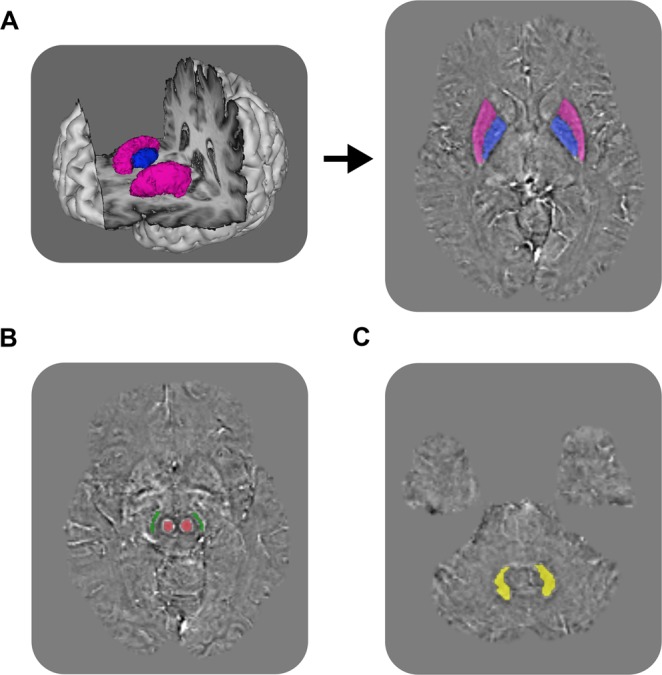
Automated segmentation of globus pallidus (blue) and putamen (magenta) on T1-weighted (A). Manual segmentation of substantia nigra (green), the red nucleus (red) (B) and the dentate nucleus (yellow) (C) on the susceptibility maps.
